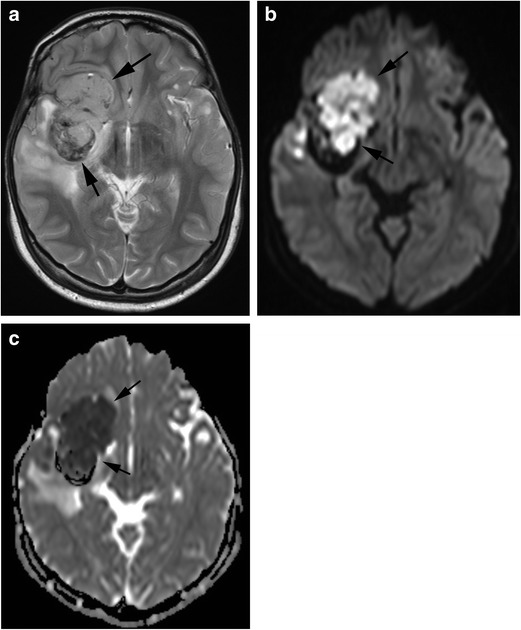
Radiation induced atypical teratoid/rhabdoid tumour. A 21-year-old man, who received radiation therapy for optic glioma during infancy, presented with headache, appetite loss and somnolence. a A T2-weighted image shows a lobulated, solid mass with internal low signal intensity, indicating a small haemorrhage in the middle cranial fossa to basal ganglia (*arrows*). b Diffusion weighted image shows hyperintensity in the tumour (*arrows*). c Apparent diffusion coefficient map shows restricted diffusion in the tumour (*arrows*)
